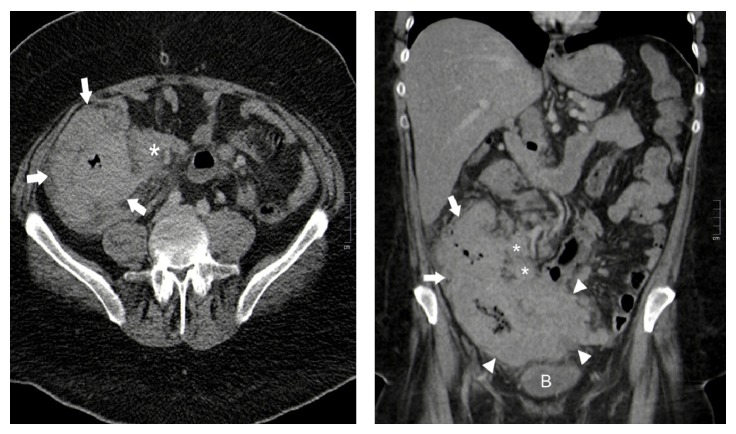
Axial and coronal CT images* through the abdomen and pelvis* from a portal venous phase whole body CT scan demonstrating marked diffuse thickening of the caecum (arrowheads) and ascending colon (arrows) with associated ileocolic lymphadenopathy (asterisks). Note the homogenous enhancement and texture of the colonic wall thickening, appearances which are more commonly seen with bowel lymphomas than bowel carcinomas. B = bladder.
